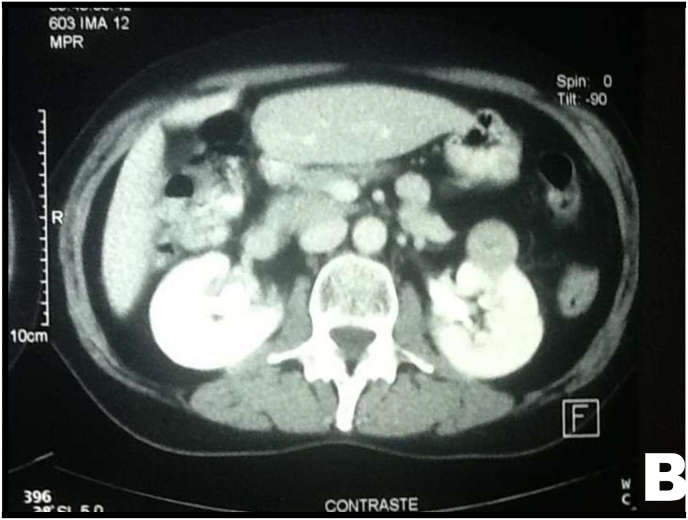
Abdominal CT showing absence of right-sided hydronephrosis and the presence of a renal mass in the right kidney.
